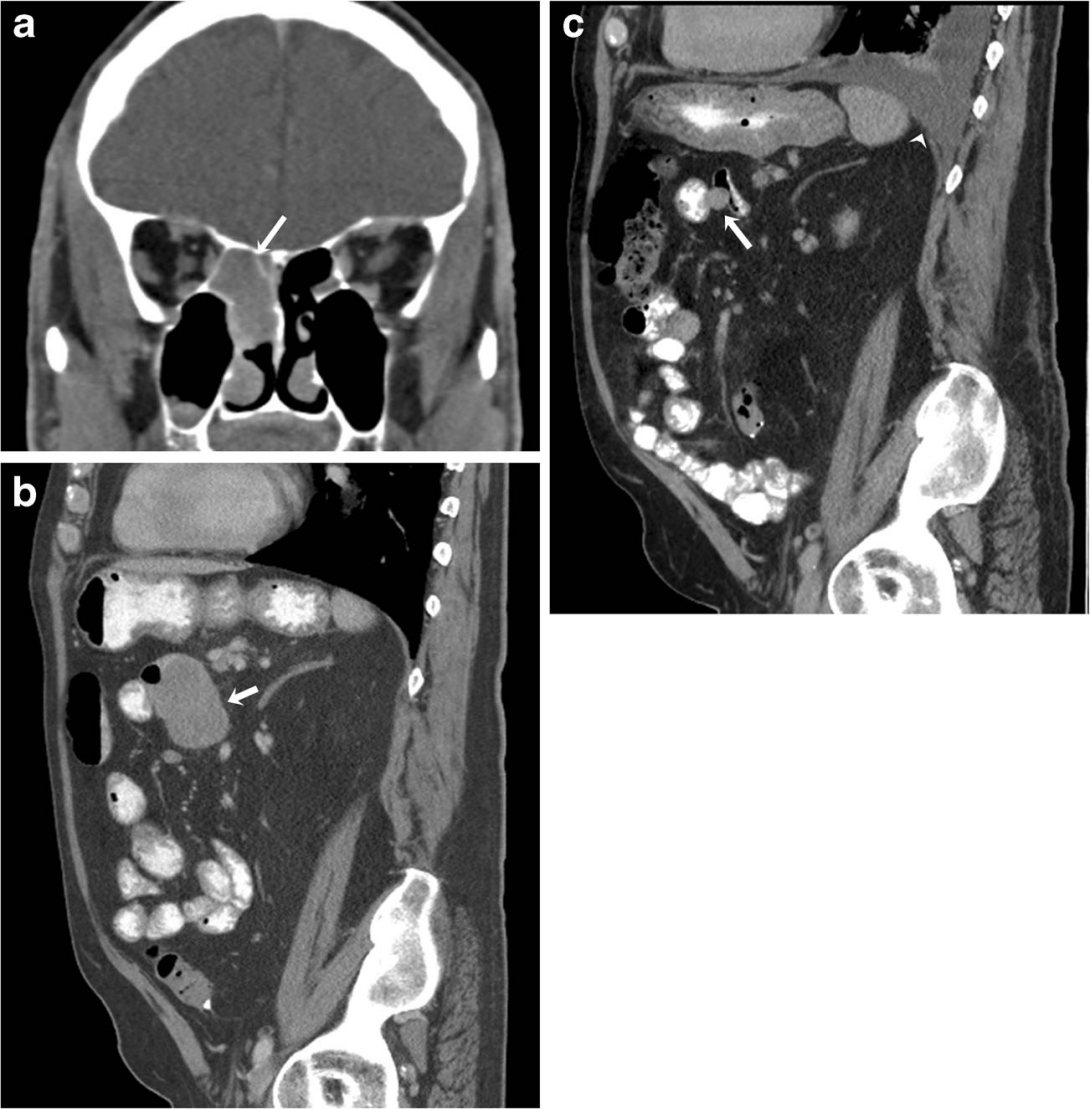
A 73-year-old male with metastatic sinonasal melanoma on treatment with BRAF and MEK inhibitors. a Coronal contrast-enhanced CT image shows a homogeneous mass lesion involving the right nasal cavity (arrow). b Sagittal contrast-enhanced CT image before the start of chemotherapy shows a large small bowel metastatic lesion (arrow). c Sagittal contrast-enhanced CT image after four cycles of chemotherapy shows a significant interval decrease in the size of the metastatic lesion and new left moderate pleural effusion (arrowhead)
